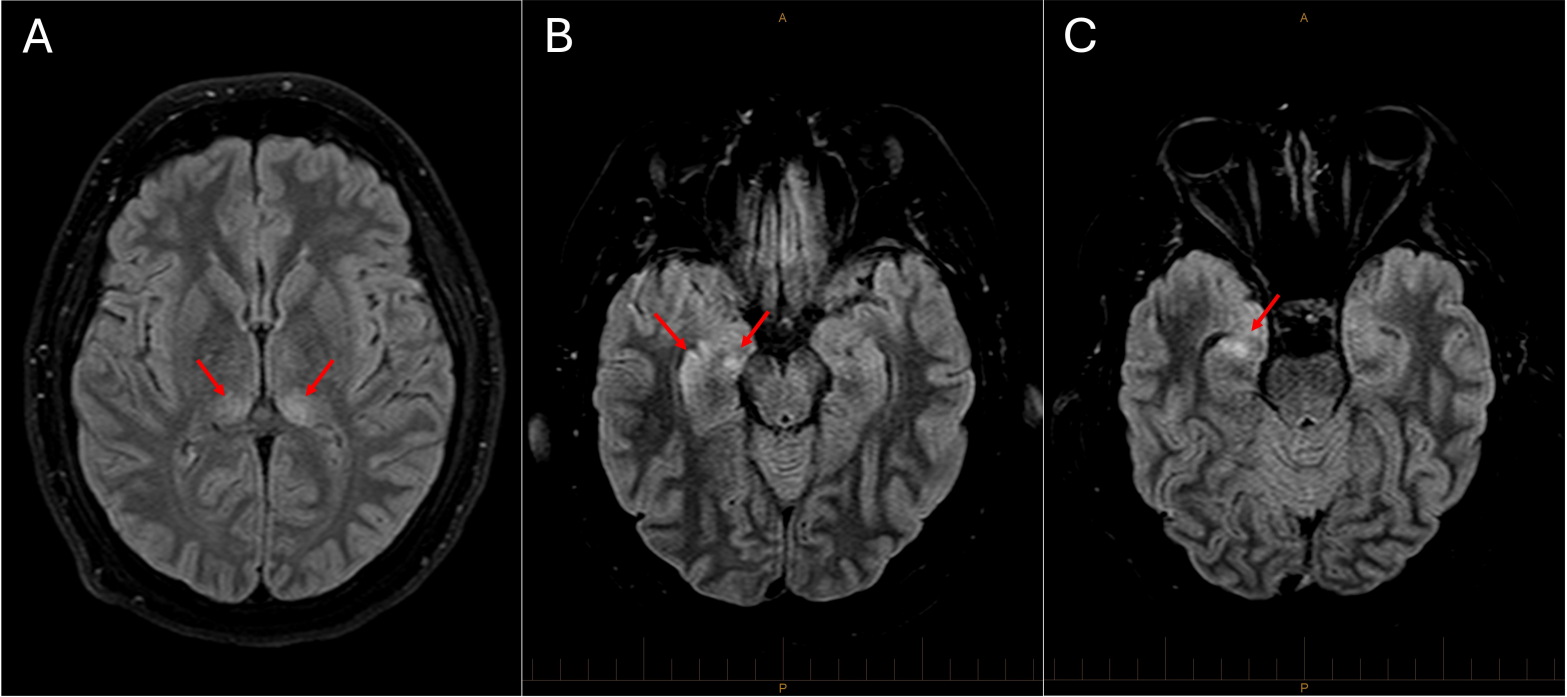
MRI of the brain showing signal hyperintensity in the bilateral posteromedial thalami (panel A) and right mesial temporal lobe structures including the hippocampus and parahippocampal gyrus (panels B and C).
